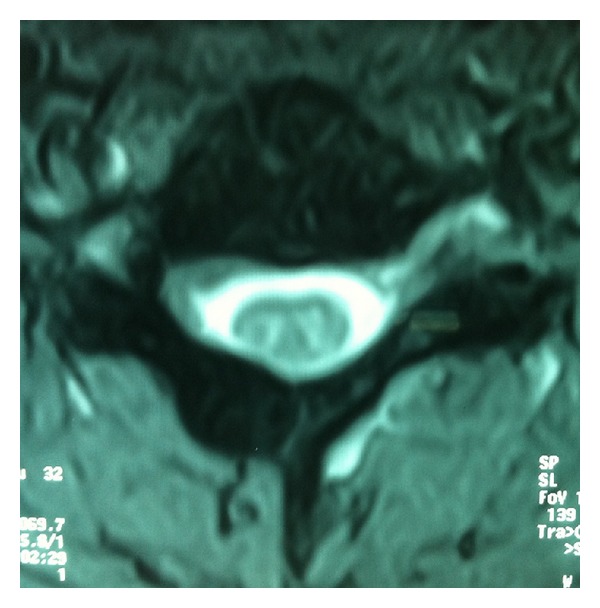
Mild expansion on the lamina of C5 vertebra and hypointense sclerotic lesion seen on T2 weighted MRI image.
